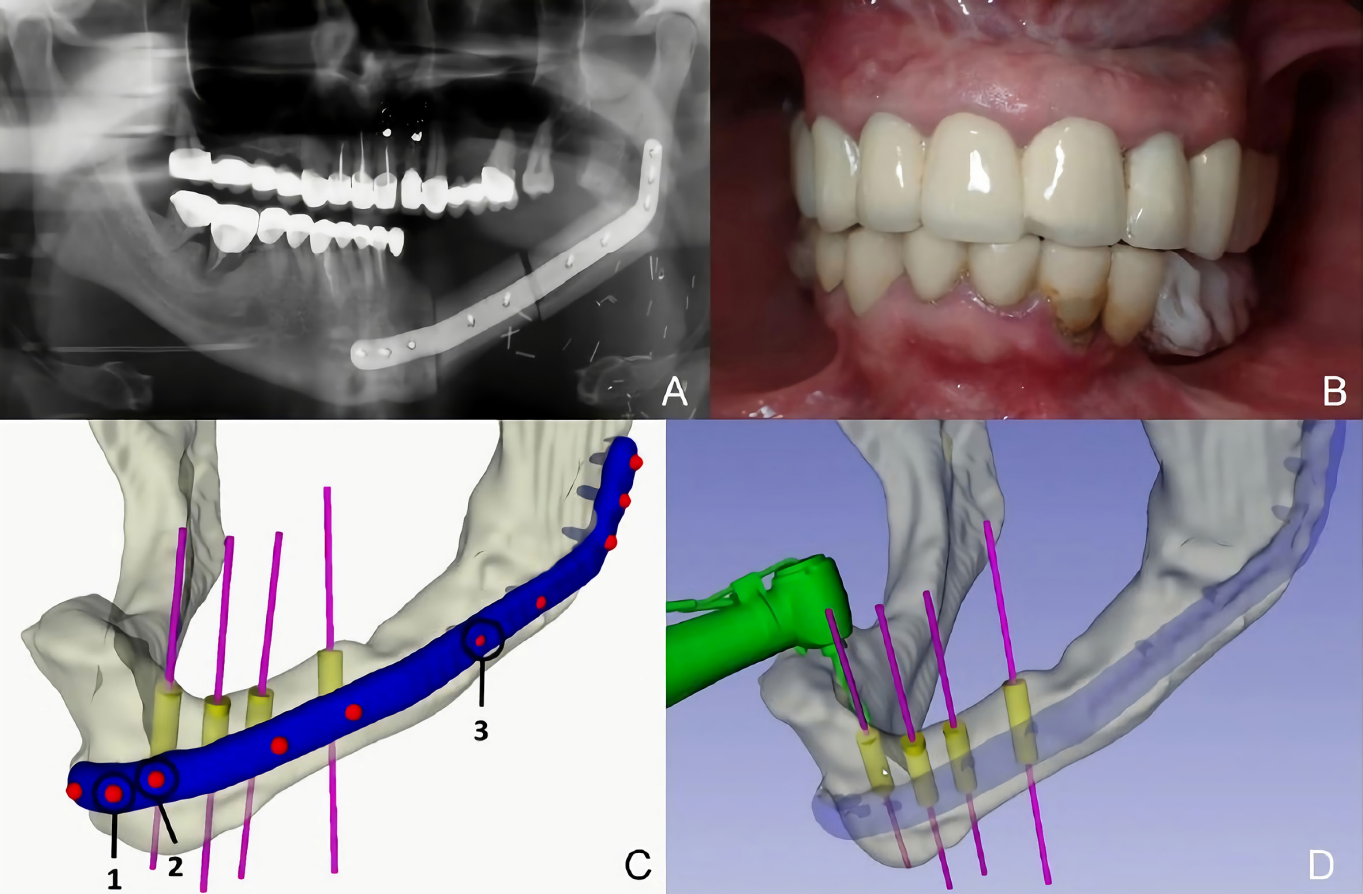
(A) Panoramic radiograph after mandibular reconstruction. (B) Splint manufactured for dynamic navigation. (C) Virtual surgical planning of 4 Ticare^®^ implants. (D) Intraoperative dynamic in-house navigation for implant placement.
